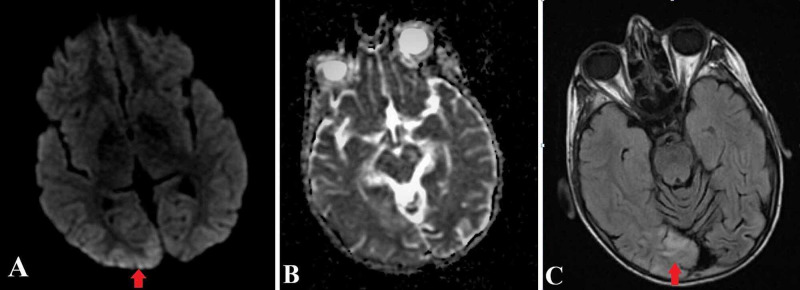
MRI sequences show restricted diffusion on DWI (A) in the right occipital region which is isodense on ADC (B) and hyperintense on T2-FLAIR (C), respectively. DWI: diffusion-weighted imaging, ADC: apparent diffusion coefficient, T2-FLAIR: T2 fluid inverted attenuation recovery.
